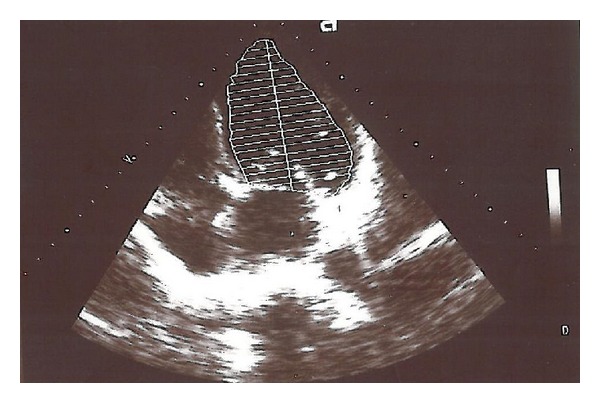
Transthoracic echocardiography revealed global hypokinesia of the LV with left ventricular ejection fraction (EF) of 20% which was measured by biplane Simpson method. The three-year-earlier echocardiography had showed ejection fraction of 50%.
